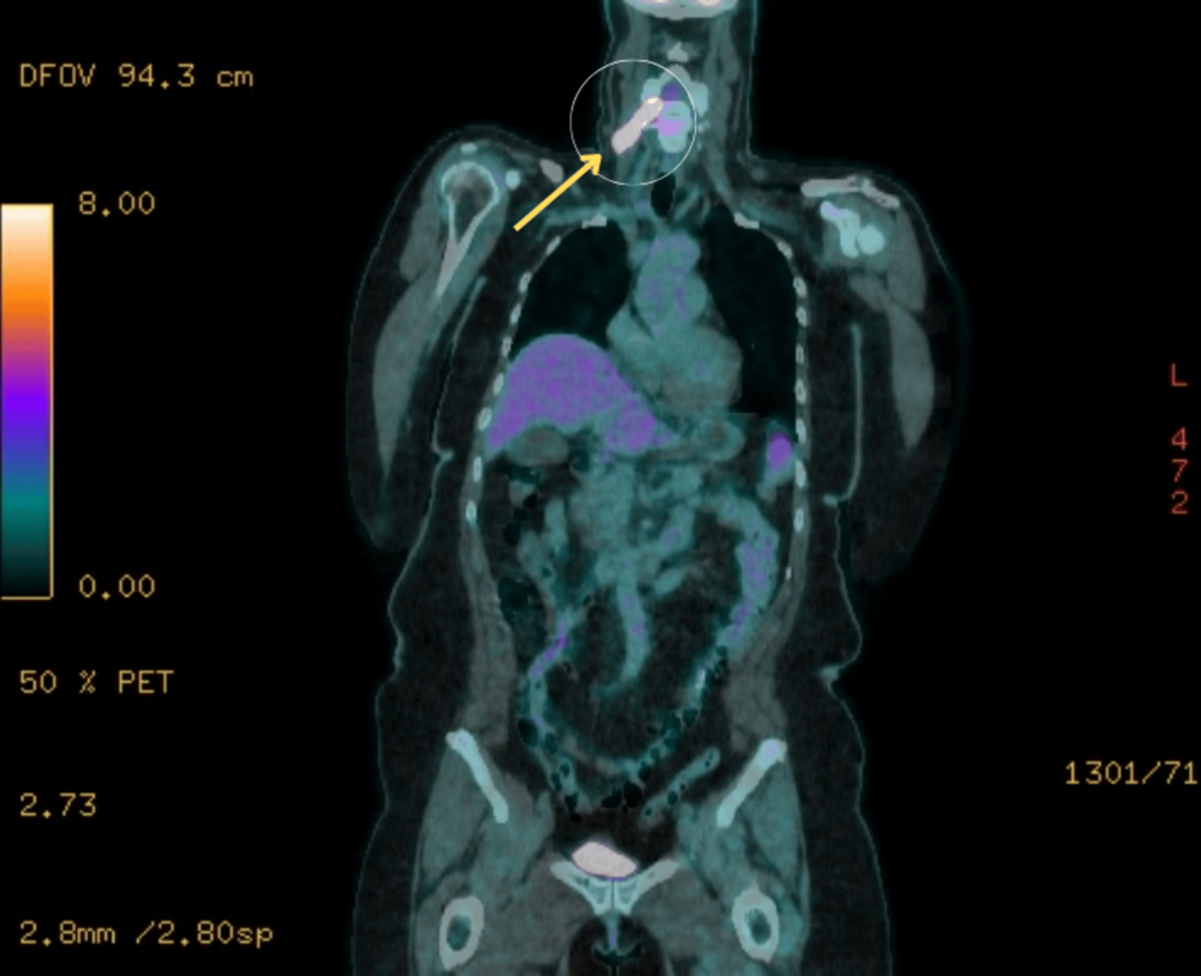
Diffuse FDG uptake along the spinal nerve roots and marrow on coronal PET-CT Coronal PET-CT image showing widespread fluorodeoxyglucose (FDG) uptake along the spinal nerve roots (yellow arrows). The diffuse metabolic activity is consistent with central nervous system involvement by lymphoma. No FDG-avid lymphadenopathy or visceral organ involvement is observed, indicating a non-nodal, extranodal pattern of disease distribution.
